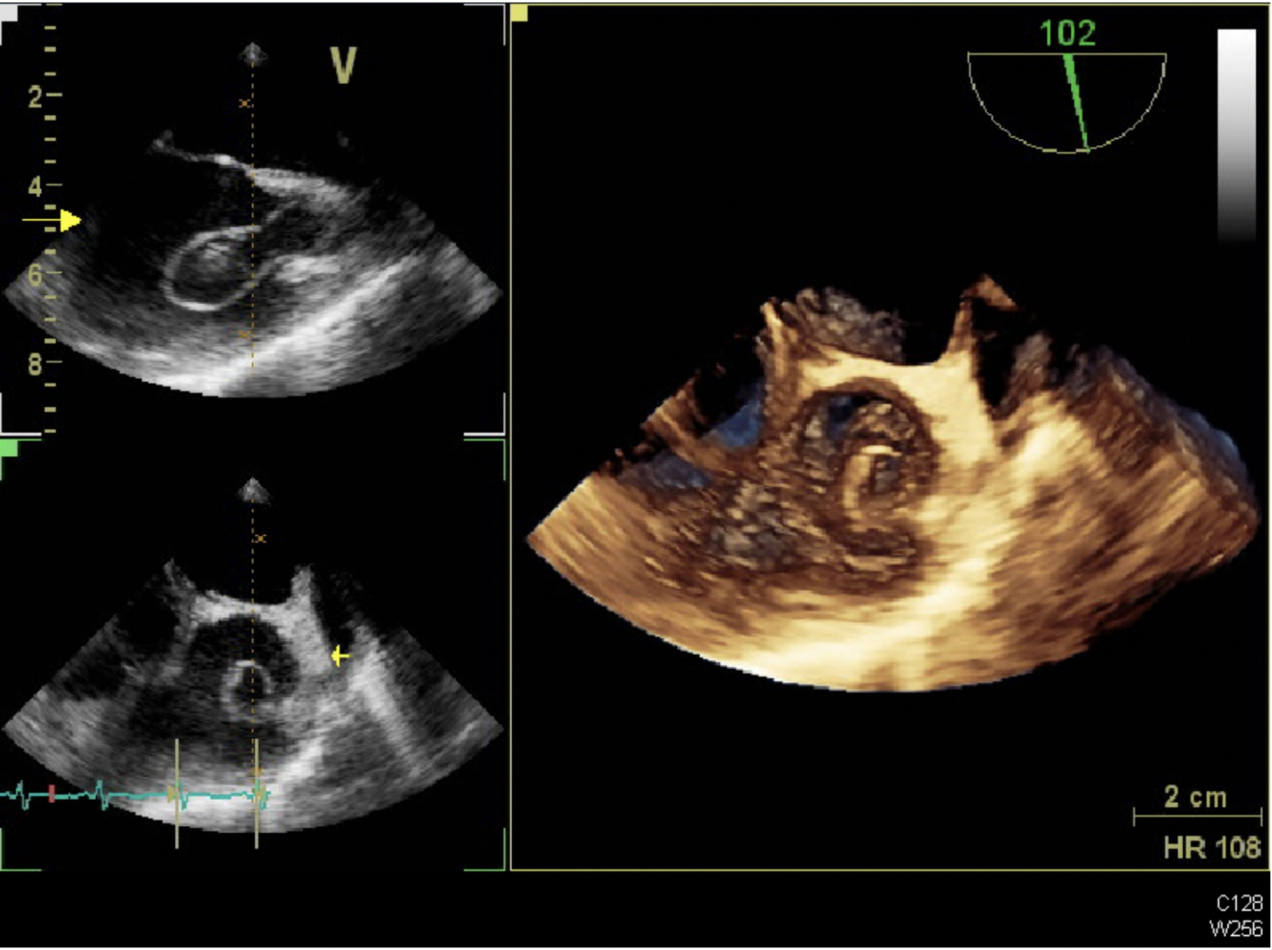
Presenting Transesophageal Echocardiogram (TEE) Imaging TEE images at presentation showing the thrombus-in-transit in the right atrium and at the atrio-caval junction
